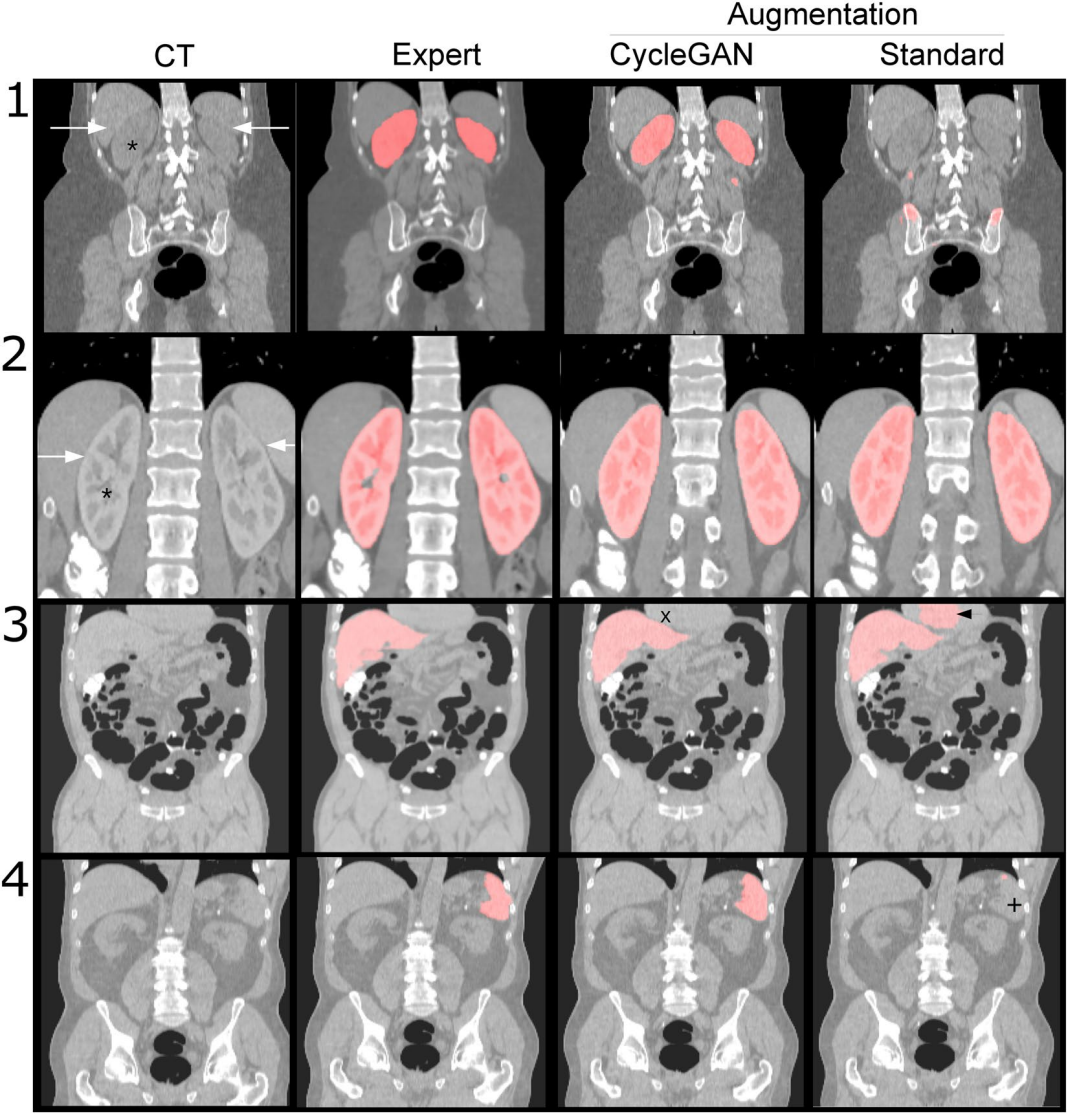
Examples of segmentations. Original CT and expert segmentation are shown in the first and second columns and CycleGAN and standard augmented training results are shown in the third and fourth columns, respectively. For detailed comments see main text.
